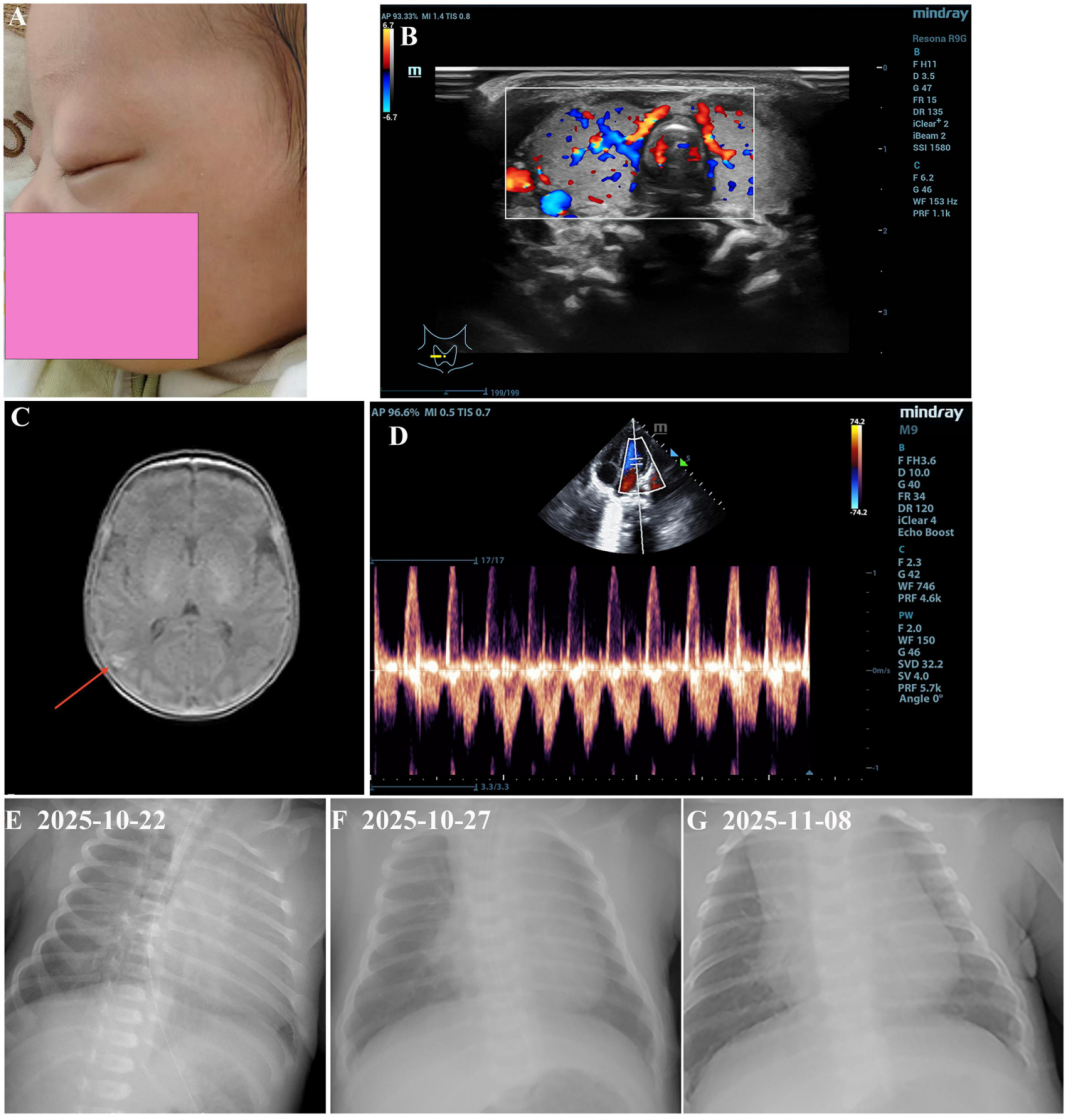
Clinical examination of abnormal indicators with neonatal hyperthyroidism. (A) Neonatal hyperthyroidism face has eyelid edema. (B) Ultrasound Examination of thyroid gland. Both thyroid glands are enlarged in shape, with smooth, clear and intact capsules. Color Doppler shows abundant blood flow signals in the gland. (C) Magnetic resonance imaging of the brain, T1W1 intensive signaling indicate an intra cerebral hemorrhages in the right occipital lobe. (D) Ultrasound examination of cardiography. Patent foramen ovale. Chest radiograph of the newborn, obtained on 22 October 2025 (E), 27 October 2025 (F), 8 November 2025 (G), showing thickened lung texture.
